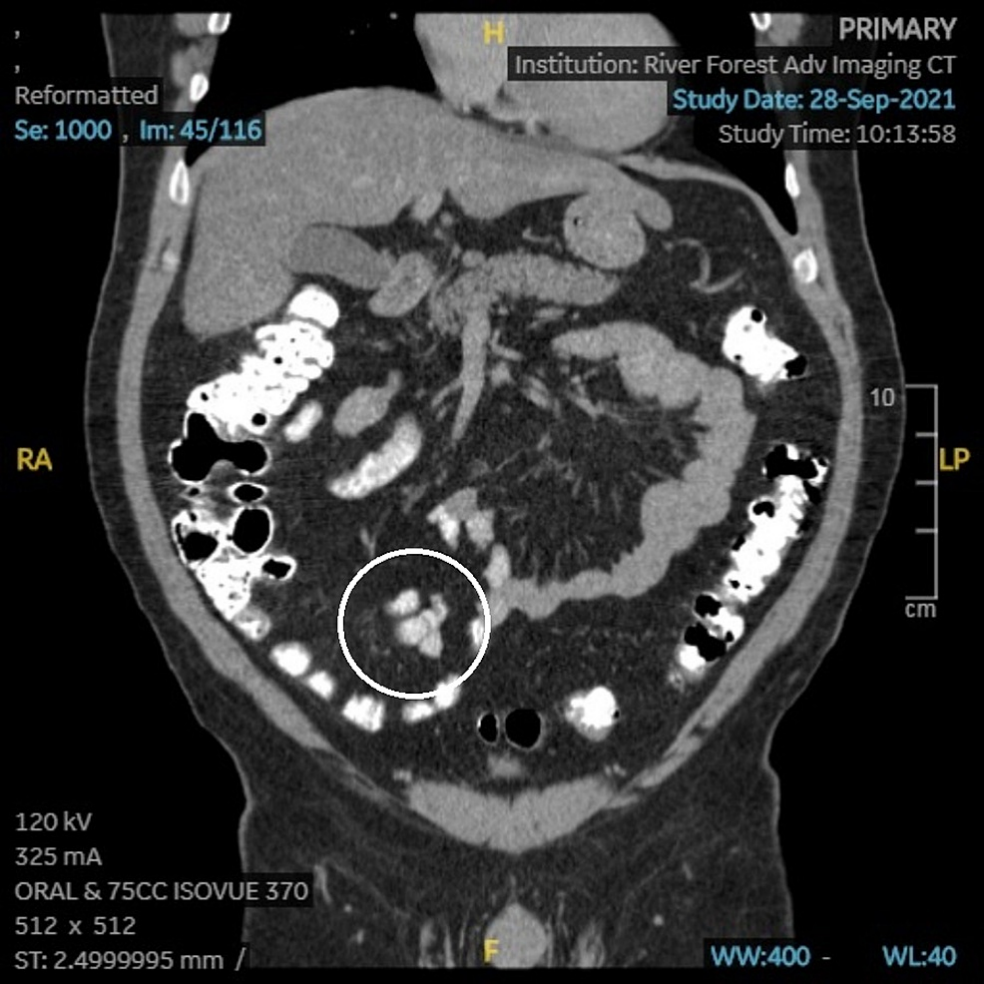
CT (coronal) image showing enlargement of the appendix and adjacent lymph nodes (circled).
